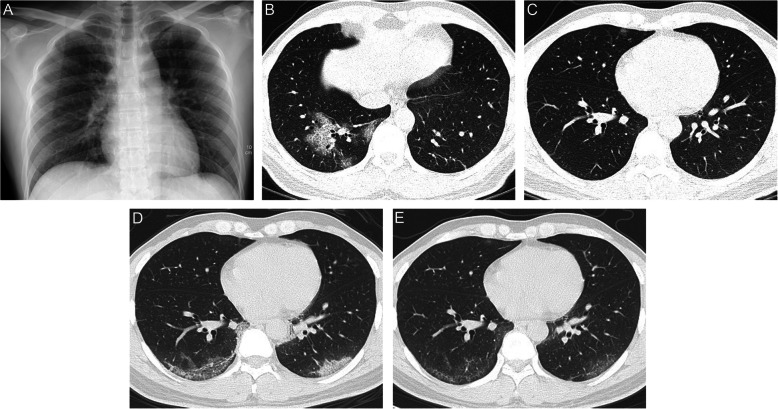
Imaging findings of the patient. a CXR showed no abnormality 5 days before admission; b and c Chest CT showed multiple GGOs in right lower lung field (admission day); d Repeat chest CT displayed larger areas of GGOs in both lower lung with a pronounced peripheral distribution imaging (day 8); e Repeat chest CT showed remission of lung lesions, with reduced density of GGOs (day 17). Abbreviation: CXR = chest X-ray, CT = computed tomography, GGOs = ground-glass opacities
